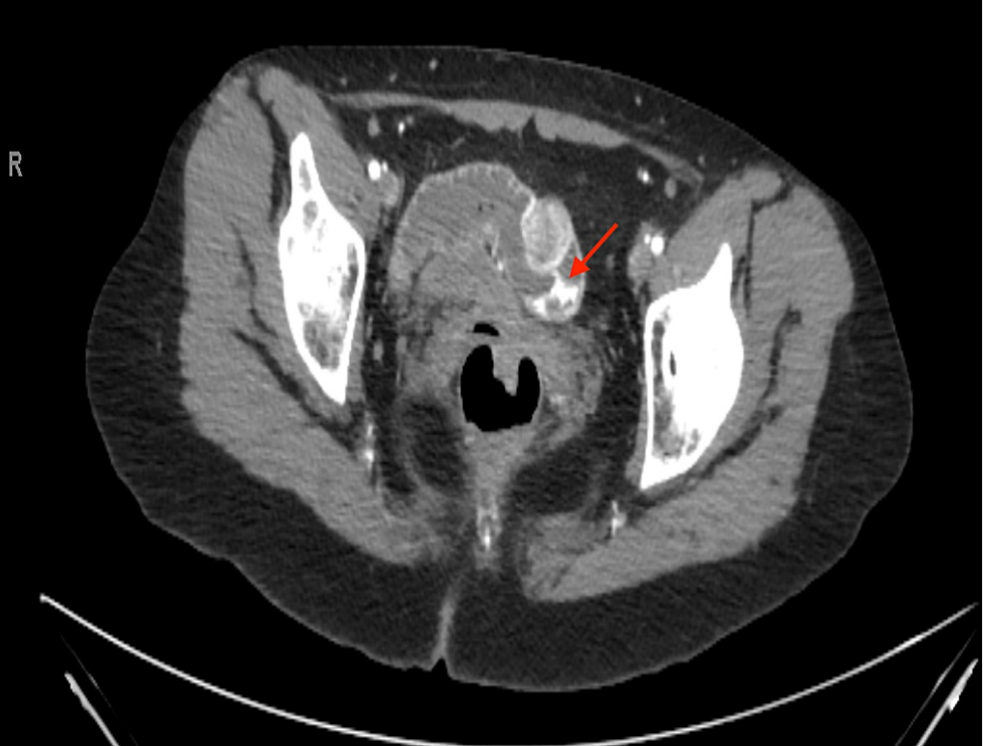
CT Abdomen with IV contrast: A lobulated endophytic avidly enhancing mass is identified in the Ileum, which measures approximately 2.4x2.2cm. This lesion demonstrates brisk active arterial bleeding into the intestinal lumen.
